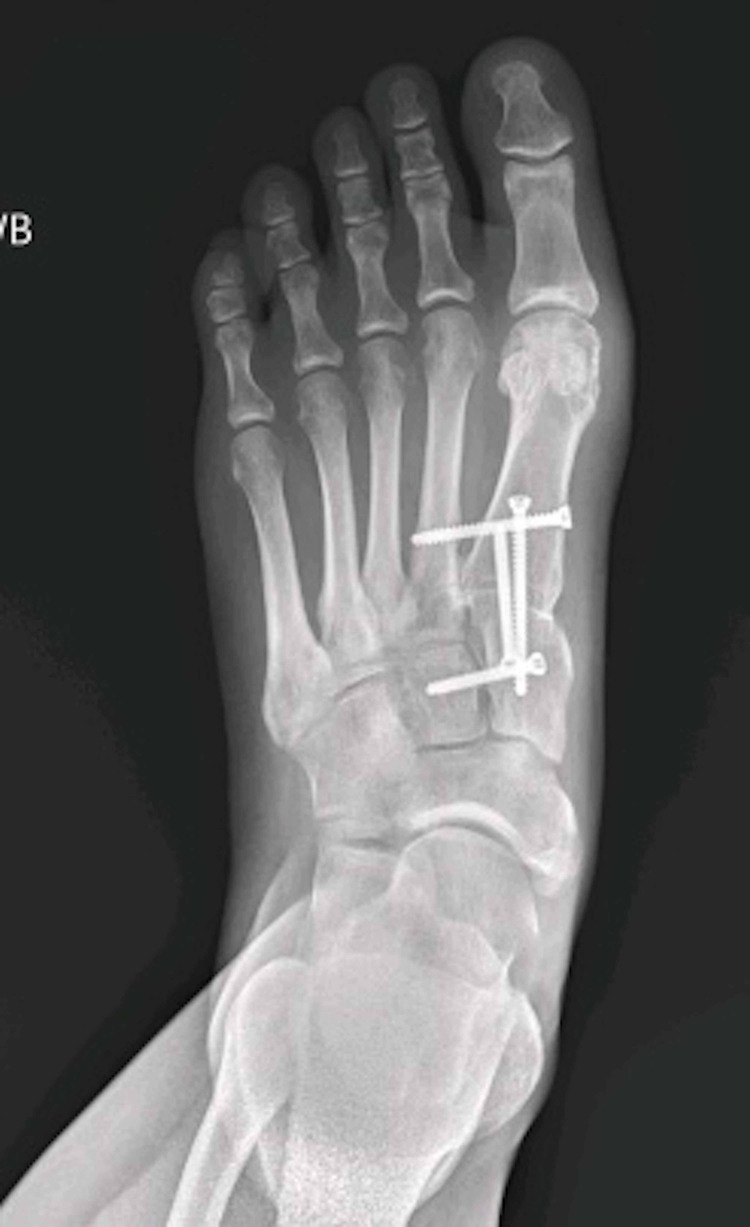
Anteroposterior radiograph demonstrating a postoperative foot from the modified Lapidus procedure.
